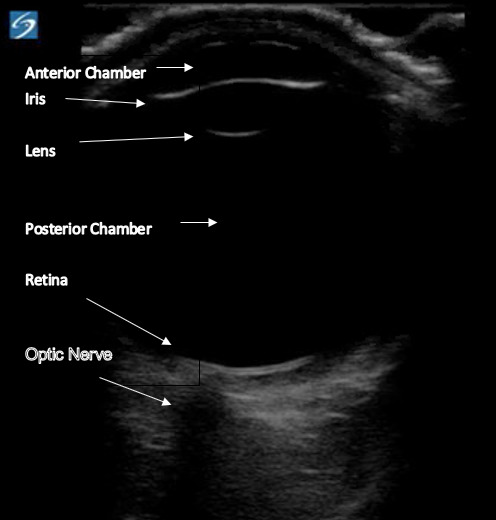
Normal ocular ultrasound with relevant anatomy.
